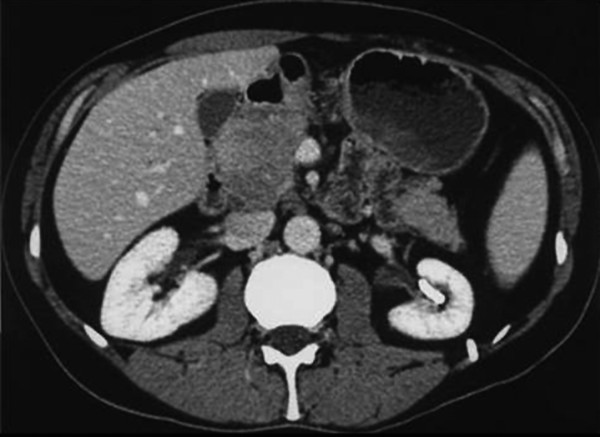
Contrast-enhanced helical CT scan. This scan demonstrates the heterogeneous enhancement of a large tumour of the head of the pancreas, in contact with the superior mesenteric vein (SMV), without signs of infiltration or encasement of the vein, or the superior mesenteric artery (SMA).
